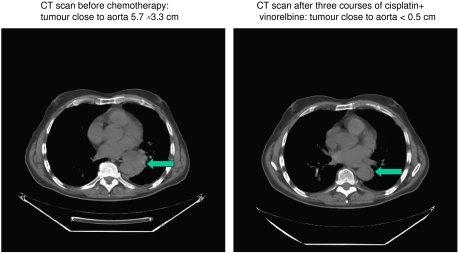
CT scan before and after three courses of cisplatin and vinorelbine in MPM with shrinkage of tumour close to aorta.
